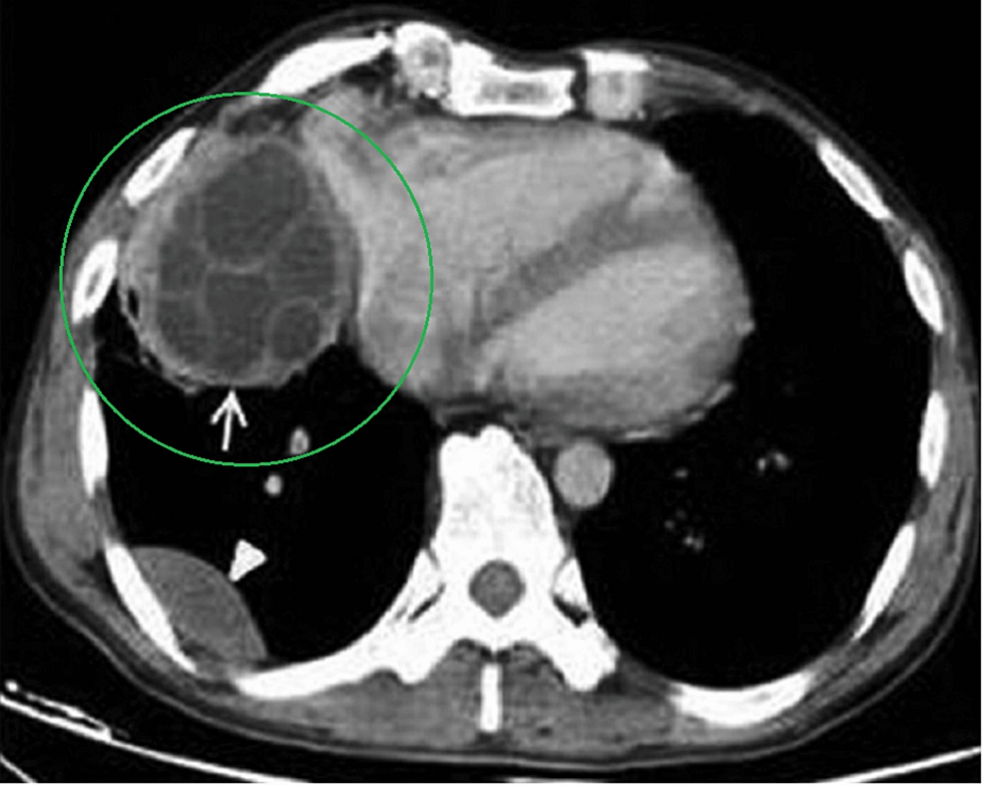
Example of an axial chest CT with contrast in a separate patient. This particular example shows cystic involvement in right cardiophrenic angle. Although these are different patients, the cysts appear similarly on both CTs with a unilocular, well-demarcated fluid density. Internal septations are present on this example due to multiple daughter cysts. Reference [8]
